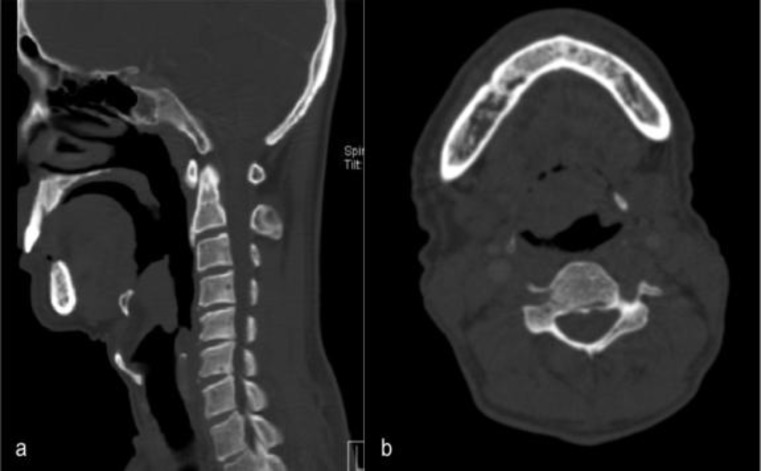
(a) Sagittal CT scan showing an epiglottic mass causing narrowing of the laryngeal inlet.  (b) Axial CT scan showing a large mass at the laryngeal aspect part of the epiglottis.
